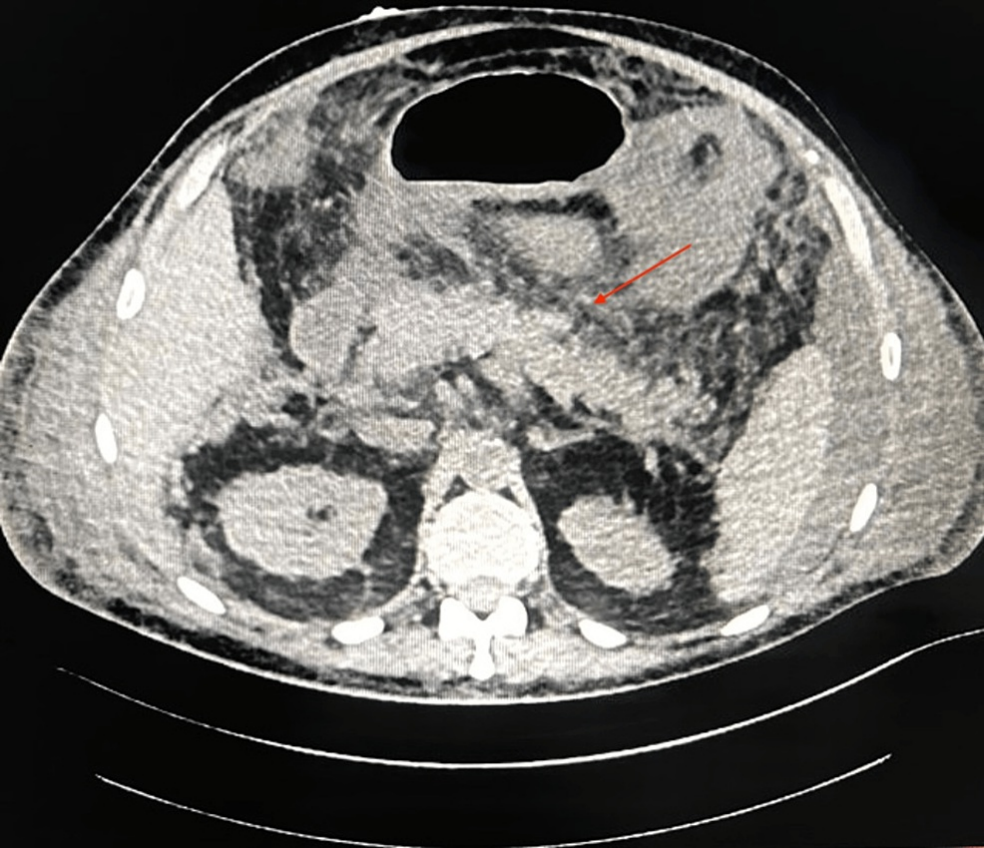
CT image showing diffuse enlargement of the pancreas with heterogenous enhancement and irregular contour of the pancreatic margins * Findings are marked with a red arrow
